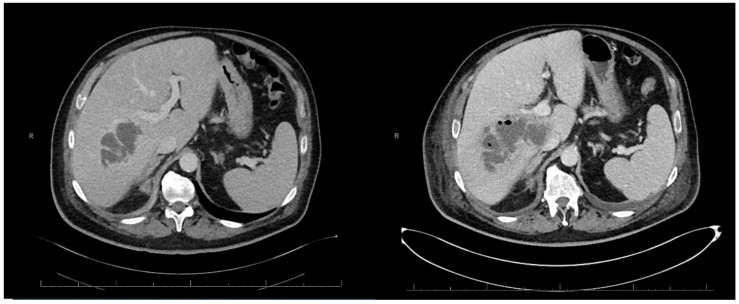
Abdomen CT scan. Temporal evolution of the intra-hepatic abscess visible in two axial sections of the first abdominal CT scans (on the left) and the second (on the right) taken 7 days apart.
